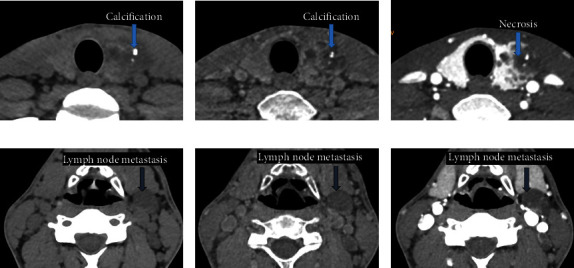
Thyroid CT images from a patient with papillary thyroid carcinoma. Compared with true noncontrast (TNC) images (a), virtual noncontrast (VNC) images (b) had a clear boundary of thyroid lesions. In displaying calcification, necrosis, and lymph node metastasis, VNC images (b and e) were consistent with TNC images (a and d). There was no enhancement of necrosis in the contrast-enhanced images (c and f).
